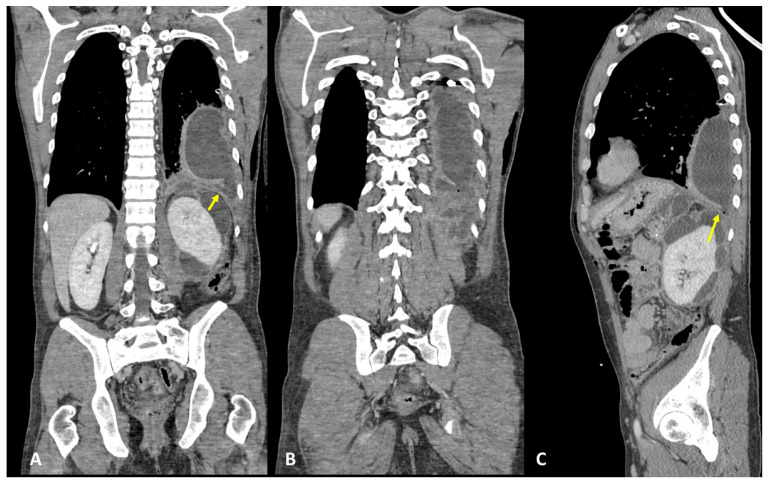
(A–C) Contrast-enhanced CT and coronal (A,B) and sagittal planes (C) show left pleural empyema with gas bubbles and multiple pancreatic pseudocysts located between the diaphragm, the pancreas tail, and the left kidney. A definite fistulous tract is seen (arrows).
